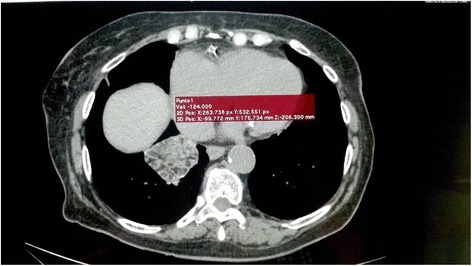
Thoracic CT 26 months after cessation of petroleum jelly
